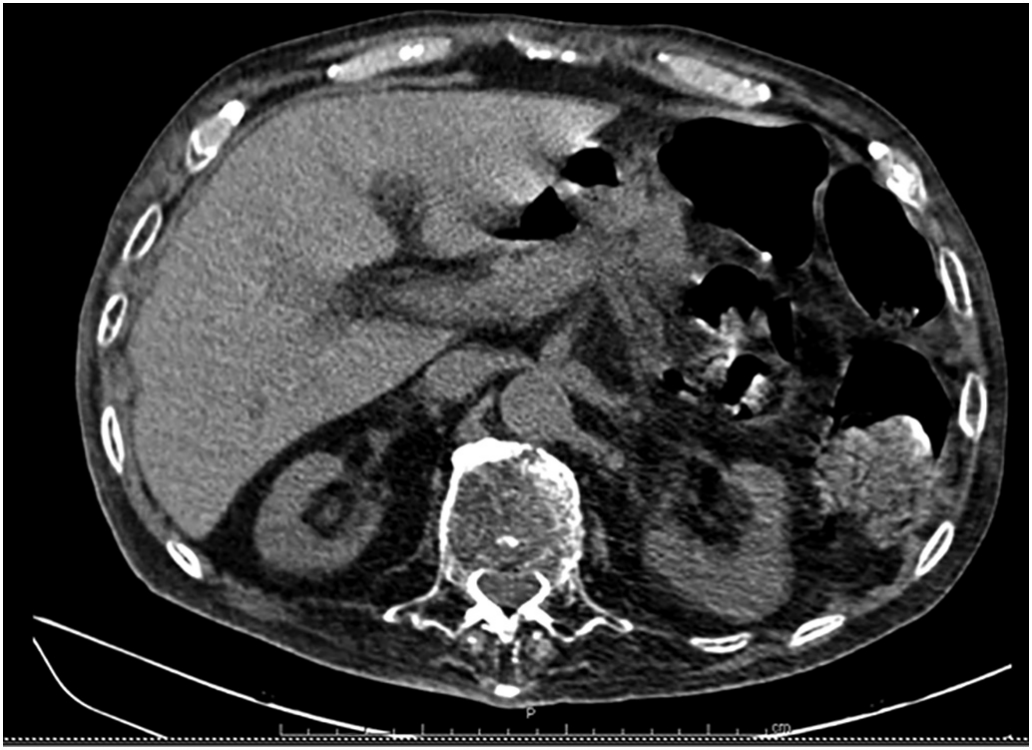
The new non-contrasted computed tomography at the same level showing no new finding compared with the previous one.
